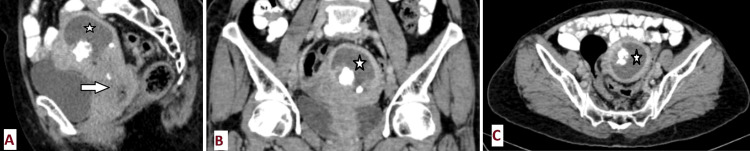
CECT abdomen and pelvis sagittal (A), coronal (B), and axial (C) sections showing heterogeneously enhancing cervical growth (arrow) involving the lower half of the uterus and the upper half of the vagina with resultant endometrial collection (star) and multiple fibroids with calcific degeneration. CECT: Contrast-enhanced CT
